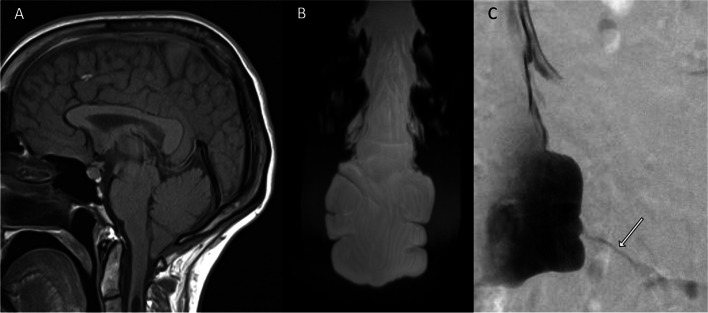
CSF-venous fistula arising from a sacral meningeal diverticulum causing intracranial hypotension. A T1-weighted sagittal image demonstrating features of intracranial hypotension including brainstem sagging, enlargement of the pituitary gland, dural venous sinus distension, and syringomyelia. B MR myelogram demonstrating a meningeal diverticulum in the sacral canal. C Digital subtraction myelogram showing a CSF-venous fistula (arrow). Case provided courtesy of Dr Wouter Schievink
